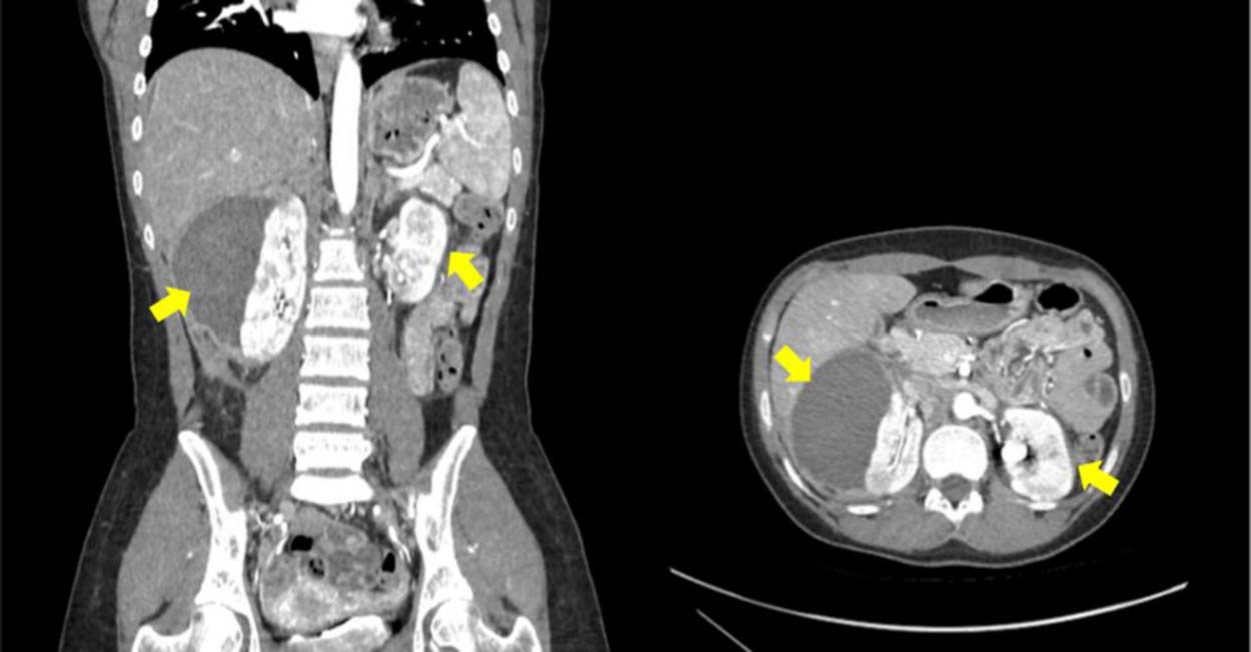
Computed tomography scan showing bilateral renal subcapsular hematoma, as well as multiple bilateral small renal aneurysms.
